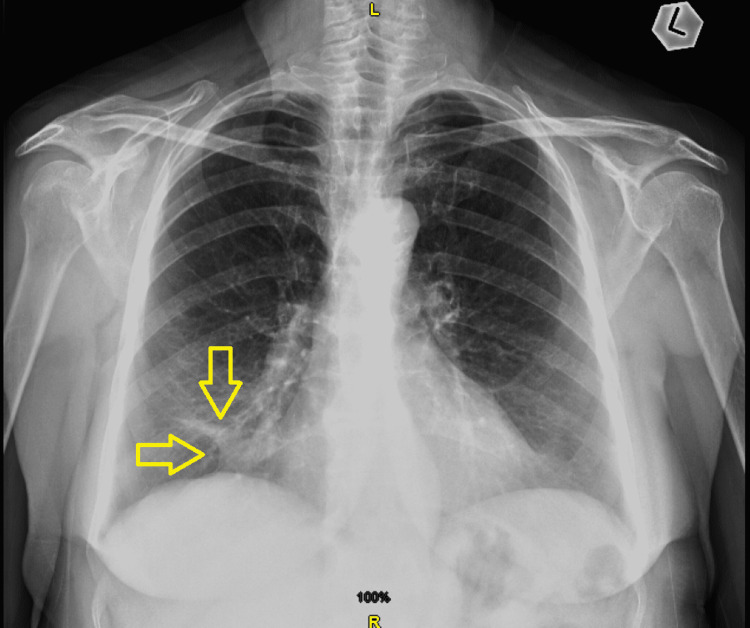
Chest X-ray (posteroanterior view) showing right pericardial triangular opacity of 2.6 x 0.9 cm with atelectatic bands
